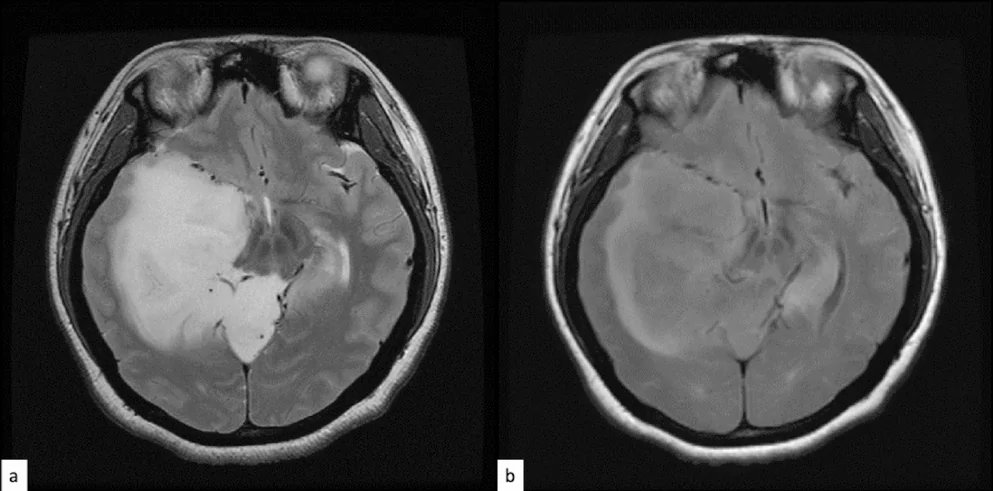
Astrocytoma, IDH-mutant, CNS WHO grade 3 in a 26-year-old woman. The tumor mass in the right temporal lobe shows hyperintensity on T2-weighted image (a) and hypointensity on fluid-attenuated inversion recovery (FLAIR) image with the peripheral hyperintense rim (b). The T2-FLAIR mismatch sign is positive in this case
